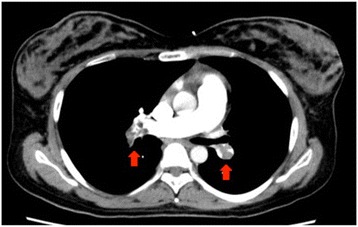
Contrast-enhanced computed tomography of the chest on admission. *Red arrows* show thrombi occupying the pulmonary arteries bilaterally
